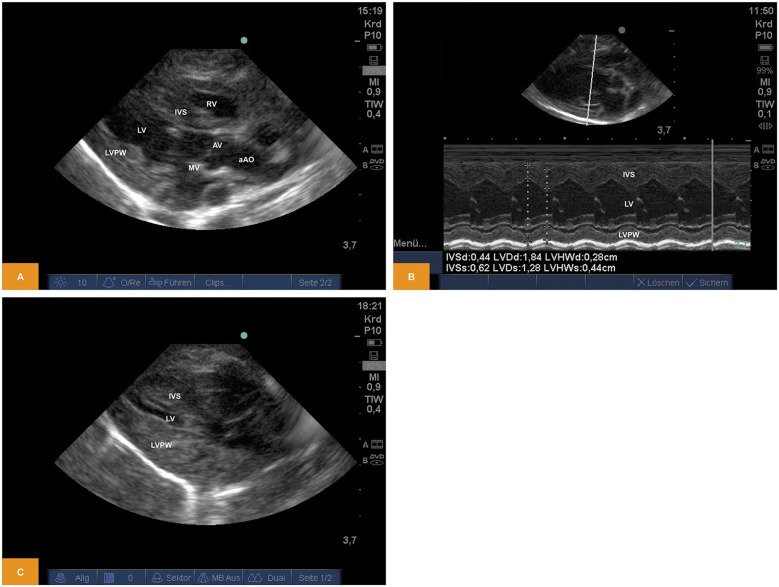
(A) Two-dimensional image of the left ventricular structures from PLAX (age: 70 h, weight: 1.45 kg). (B) M-Mode of the left ventricle obtained from PLAX (age: 70 h, weight: 1.45 kg). (C) Two-dimensional image of the left ventricular structures from PLAX after blood loss of approximately one third of blood volume (age: 12 h, weight: 1.40 kg). aAo, ascending aorta; AV, aortic valve; IVS, interventricular septum; LV, left ventricle; LVPW, left ventricular posterior wall; MV, mitral valve; PLAX, parasternal long axis; RV, right ventricle.
